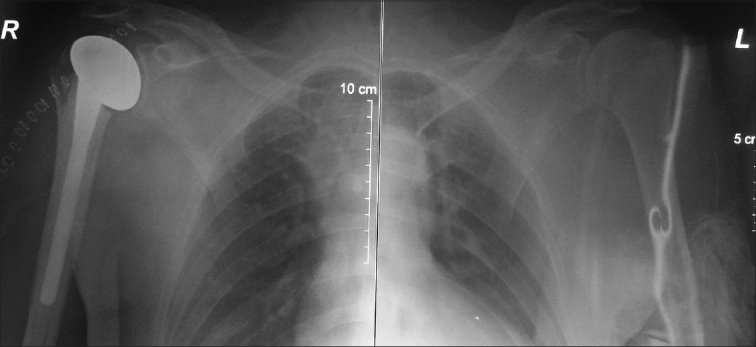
Postoperative roentgenograms of both shoulders
